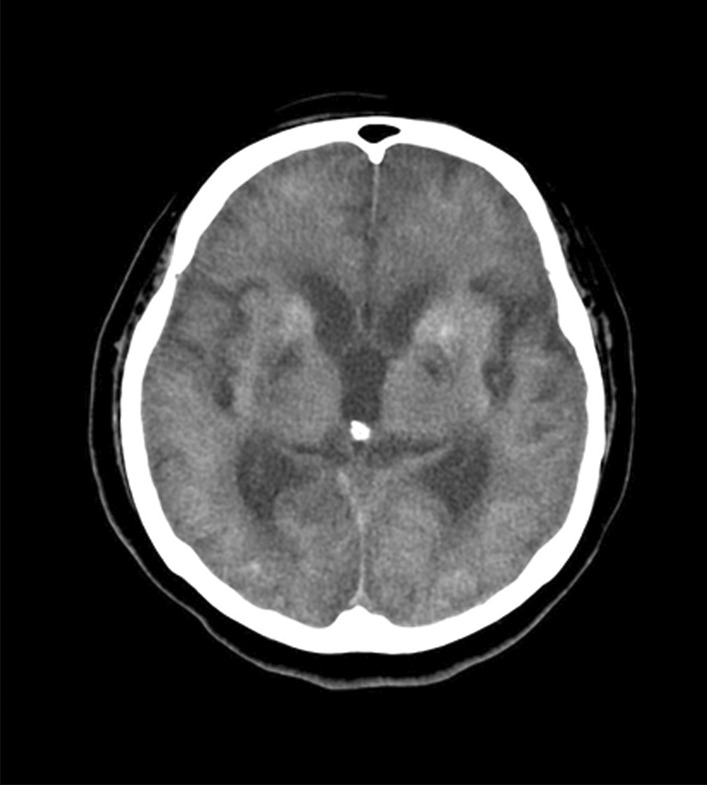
Computed tomography images of the head. Images of the whole brain indicated hypoxic encephalopathy
